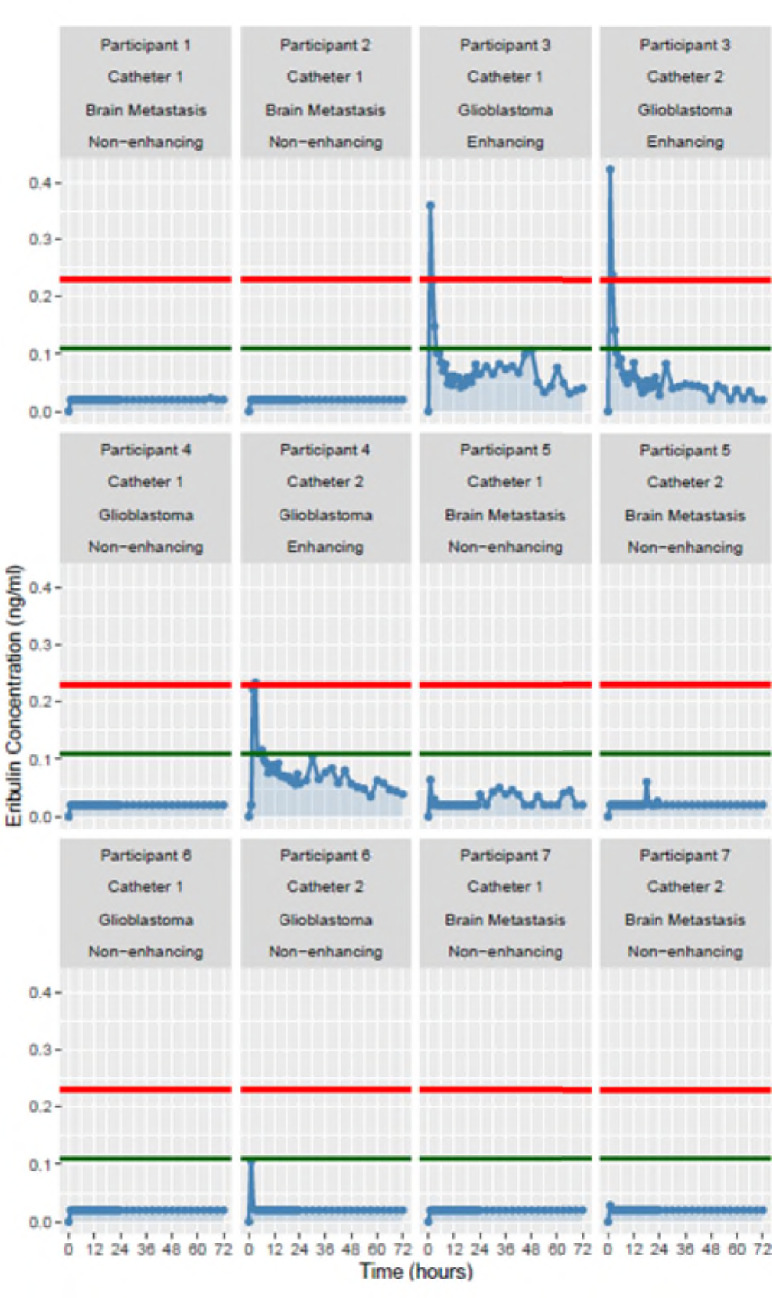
Summary of erbulin brain concentrations in study participants obtained by intracerebral microdialysis. “Non-enhancing” and “enhancing” refer to the radiographic appearance on T1 post-contrast MRI of brain tissue type (area of intact versus disrupted blood–brain barrier, respectively) in which the catheter tip was placed. The colored horizontal lines on each panel represent previously reported in vitro IC_50_ values for eribulin against two different human glioblastoma cell lines (U251; green and U87; red). [5]
